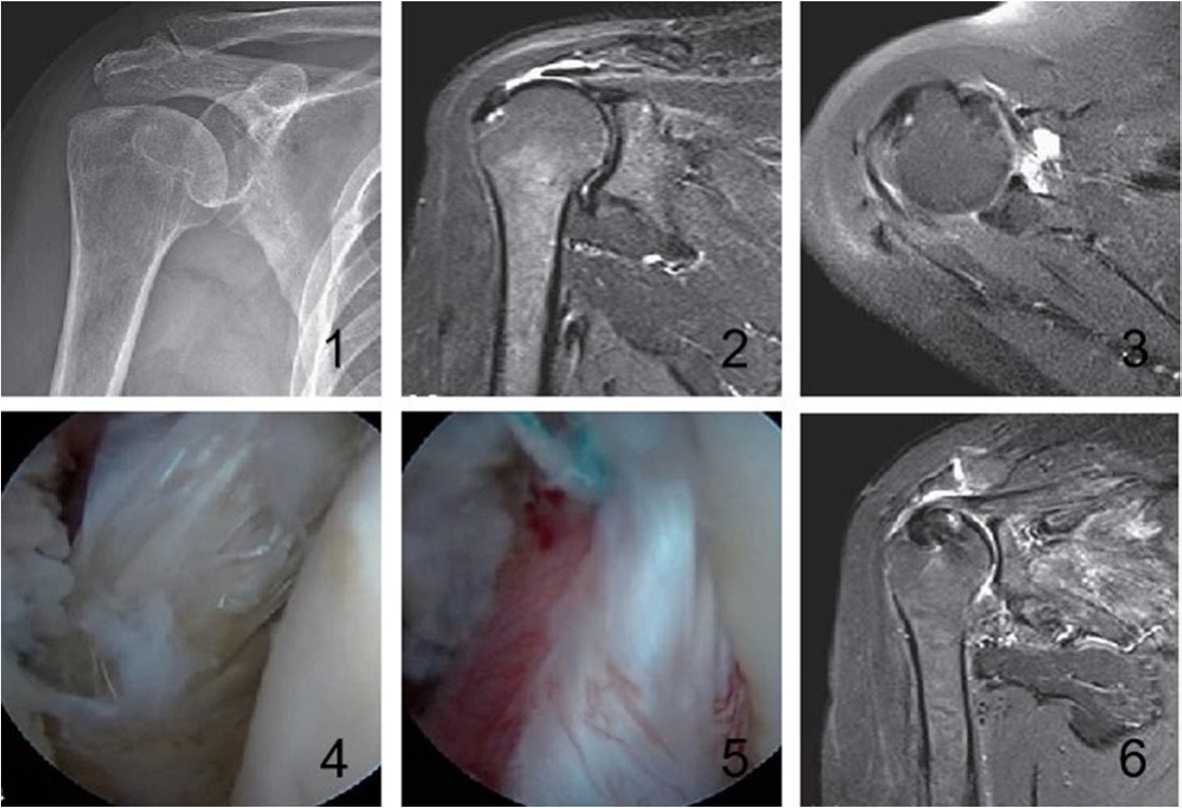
Note: 2–1: preoperative anteroposterior radiograph shows increased bone density of the acromion and greater tuberosity; 2–2: Preoperative oblique MRI T2WI of the shoulder joint shows supraspinous tendon tear, Patte stage 2 retraction; 2–3: Preoperative transverse MRI T2WI of the shoulder joint reveals mixed hyperintensities in the subscapularis tendon; 2–4: Intraoperative arthroscopy shows injury in the upper 1/3 of the subscapularis tendon (Lafosse I); 2–5: Intraoperative arthroscopy demonstrates subscapularis tendon Lafosse I injury. Repair of the subscapularis tendon was undertaken; 2–6: MRI T2WI in the oblique view at the final follow-up visit reveals an anchor in the greater tuberosity of the humerus and continuous recovery of the supraspinatus tendon
